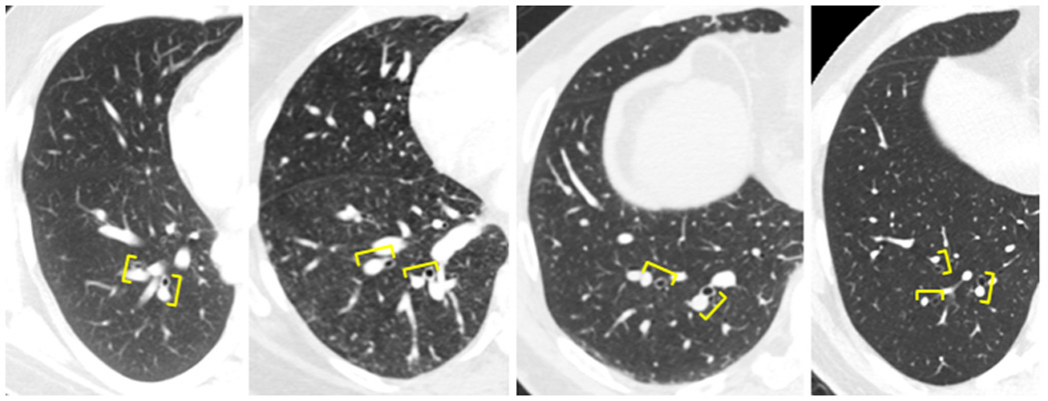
Axial CT images of the right lower zone in a patient with hepatopulmonary syndrome (A), subclinical hepatopulmonary syndrome (B), liver dysfunction only (C), and control subject (D) with representative artery and accompanying bronchus highlighted (brackets).
